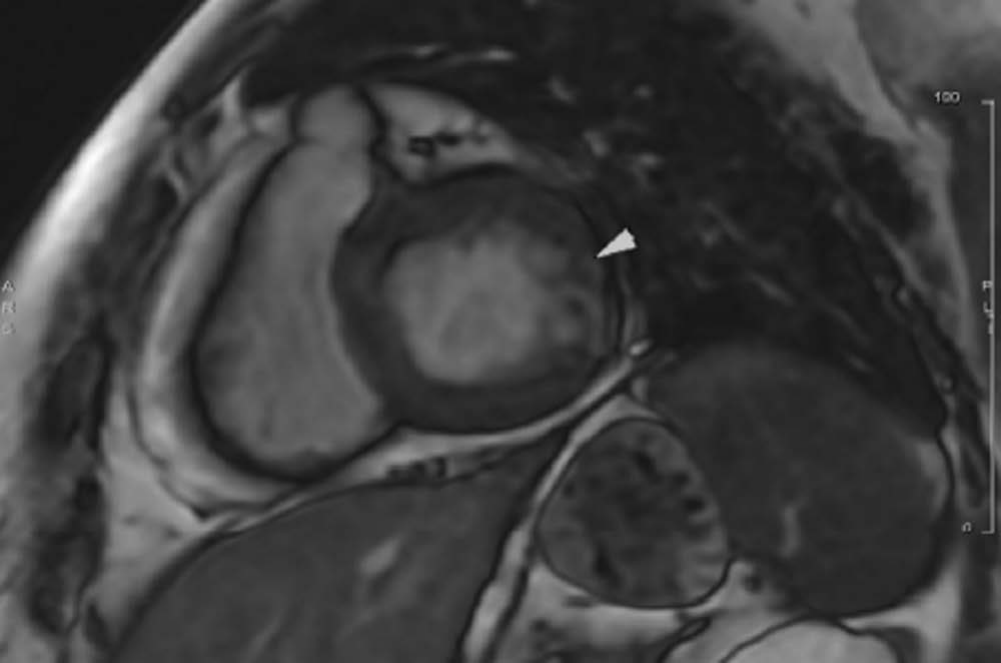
Cardiac MRI at 3 weeks after hospitalization showed patchy intramyocardial late gadolinium enhancement at basal anterolateral.
